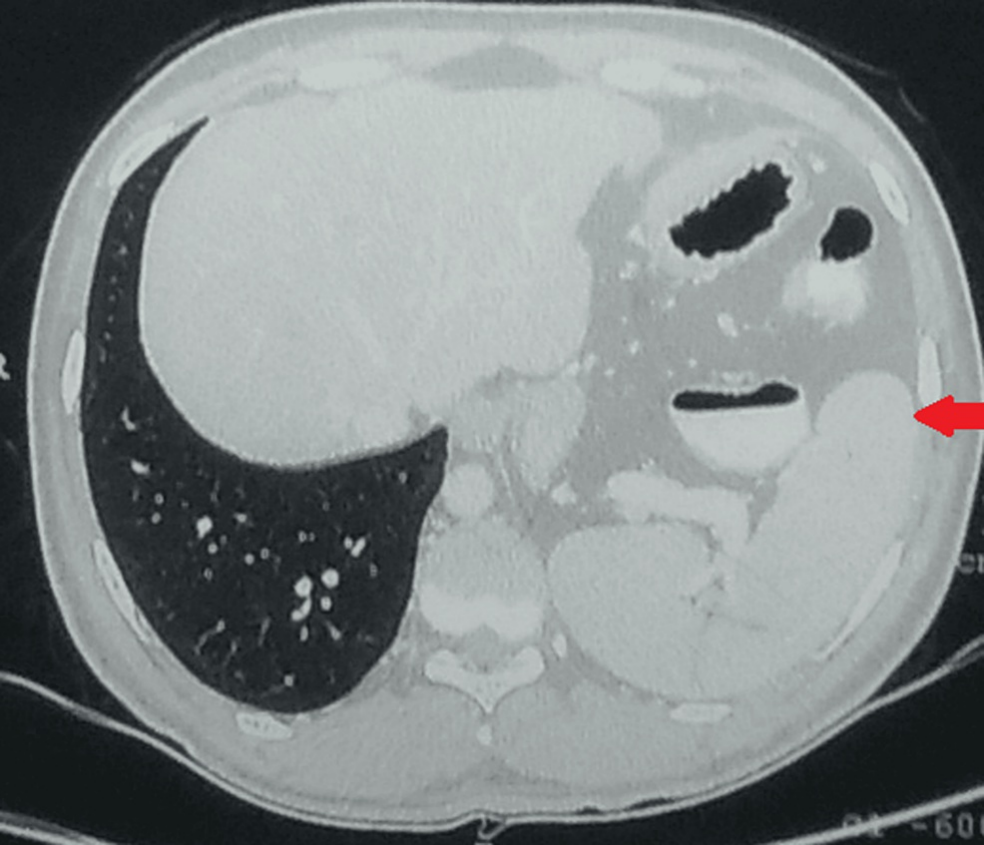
Contrast-enhanced CT of the chest. Arrow suggestive of a raised left hemidiaphragm with a superiorly displaced spleen, stomach, and splenic flexure of the colon, causing a mass effect on the ipsilateral lung, carina, and pushing the heart towards the right.
